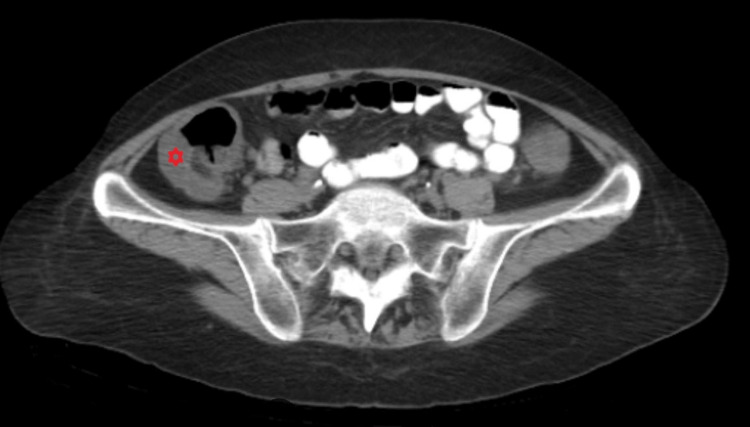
CT scan shows diffuse wall thickening and edema of colon from the right hemicolon to the rectum.
